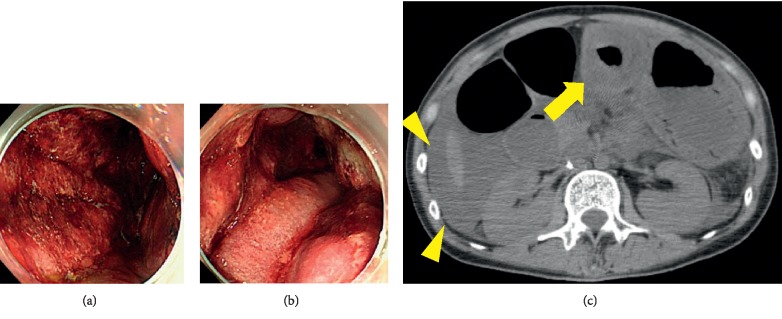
Phlegmonous gastritis. (a, b) Upper endoscopy. The gastric mucosa was reddish, and the folds were markedly thickened. (c) Abdominal CT. Fluid level was noted in the stomach. Arrow, thickened wall of the antrum and the pylorus with hypodense areas inside. Arrowheads, ascites.
